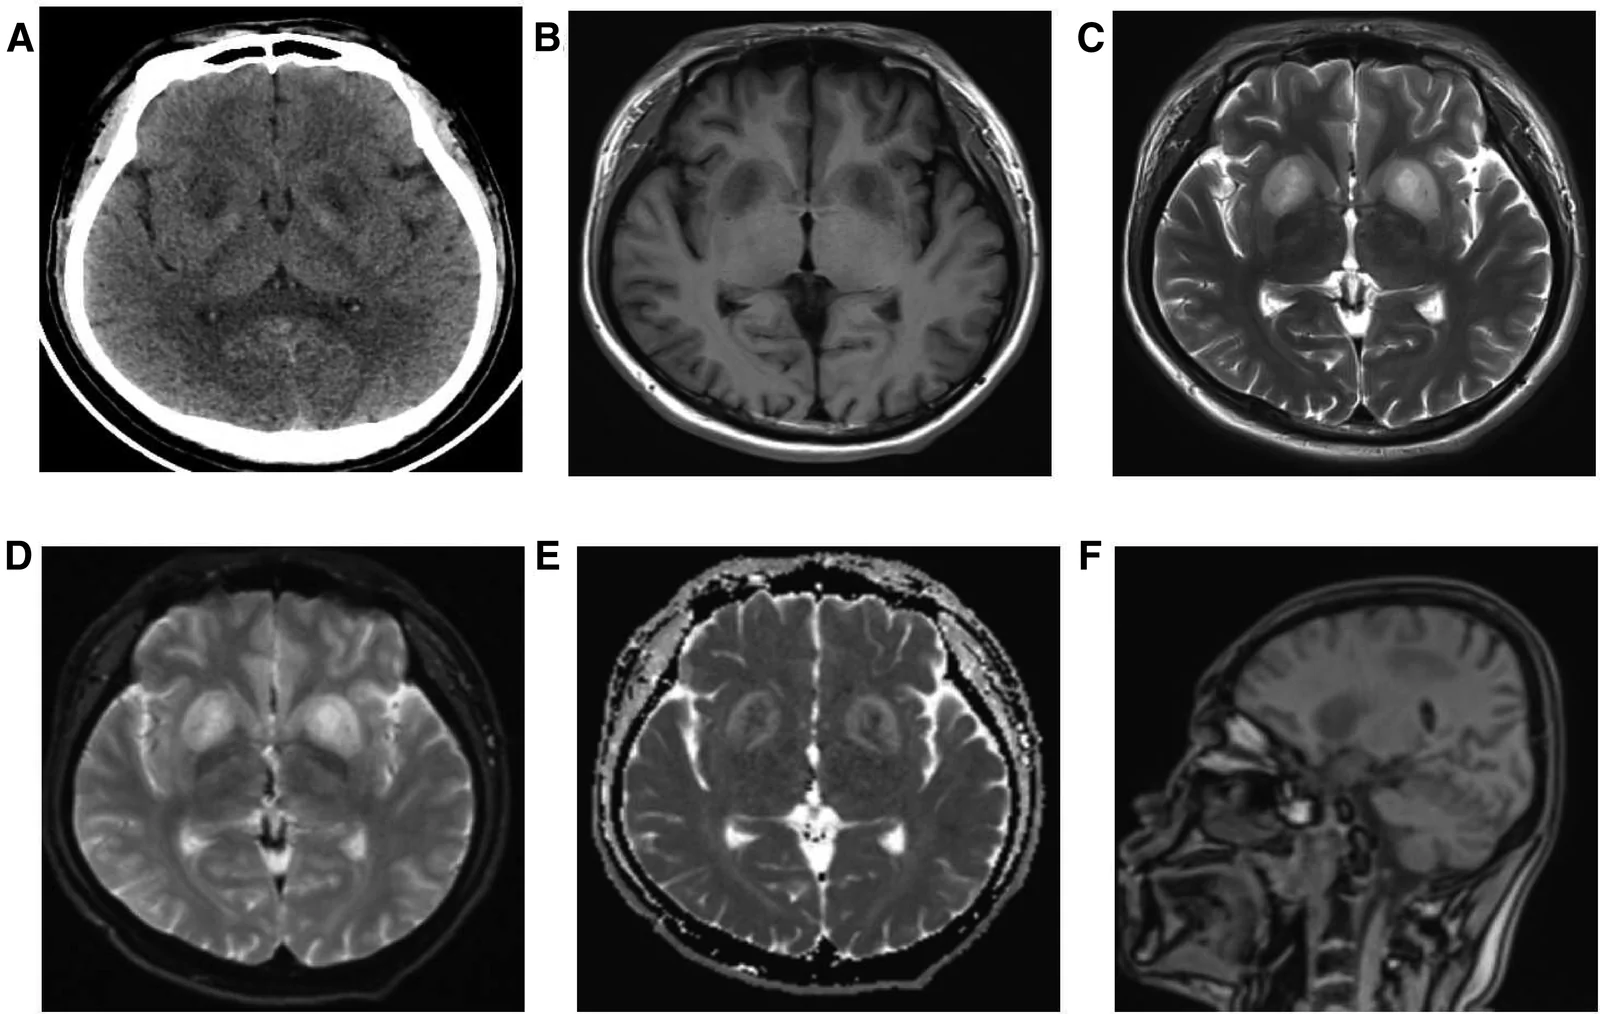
Neuroimaging findings in Case 2. (A) Brain computed tomography (CT) demonstrated bilateral lesions involving the lenticular and caudate nuclei. (B) Coronal T1-weighted image (T1WI), (C) coronal T2-weighted image (T2WI), (D) diffusion-weighted imaging (DWI), and (E) apparent diffusion coefficient (ADC) map confirmed bilateral symmetrical involvement of the lenticular and caudate nuclei. (F) Sagittal T1-weighted image demonstrated persistent lesions involving the basal ganglia.
